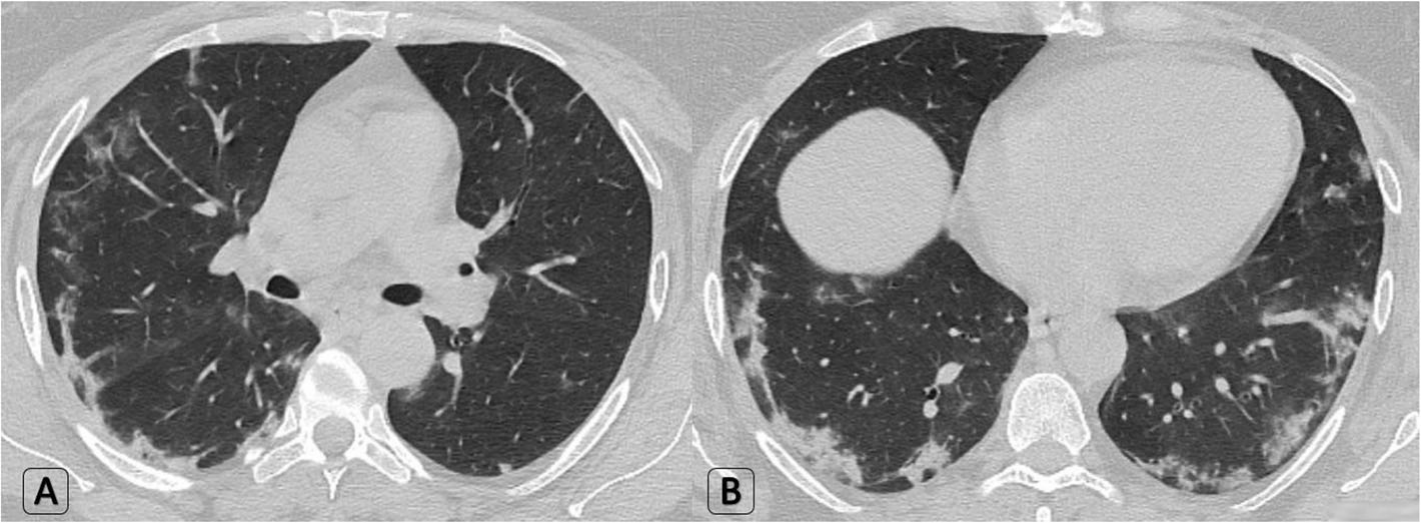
A 42-year-old male patient known for COVID-19: Follow-up HRCT scans performed 2 weeks after announcing recovery. Patient was completely asymptomatic. O_2_ saturation = 97%. Unremarkable laboratory results. a-b Axial HRCT lung window showed residual bilateral sub-pleural curvilinear fibro-consolidative patches. Clinical decision was no need for re-isolation or re-hospitalization
